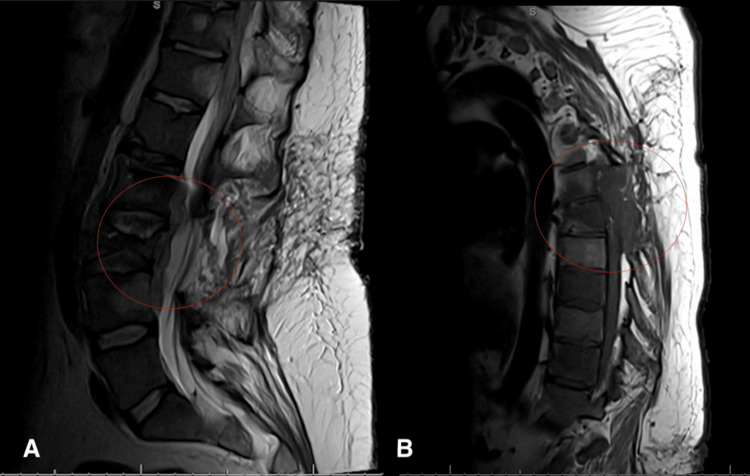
Sagittal T2-weighted magnetic resonance imaging demonstrating progressive metastatic epidural disease with multilevel spinal canal stenosis Sagittal T2-weighted MRI of the thoracic and lumbar spine obtained in December 2025, demonstrating interval progression of metastatic disease with epidural involvement compared with prior imaging. (A) Sagittal T2-weighted lumbar spine image demonstrating a pathologic compression fracture of the L3 vertebral body with associated expansile soft-tissue mass extending into the epidural space, resulting in moderate to severe spinal canal stenosis and thecal sac compression. (B) Sagittal T2-weighted thoracic spine image demonstrating an epidural mass at the T6-T7 level within the posterior spinal canal, resulting in significant spinal canal narrowing consistent with metastatic epidural disease MRI: magnetic resonance imaging
